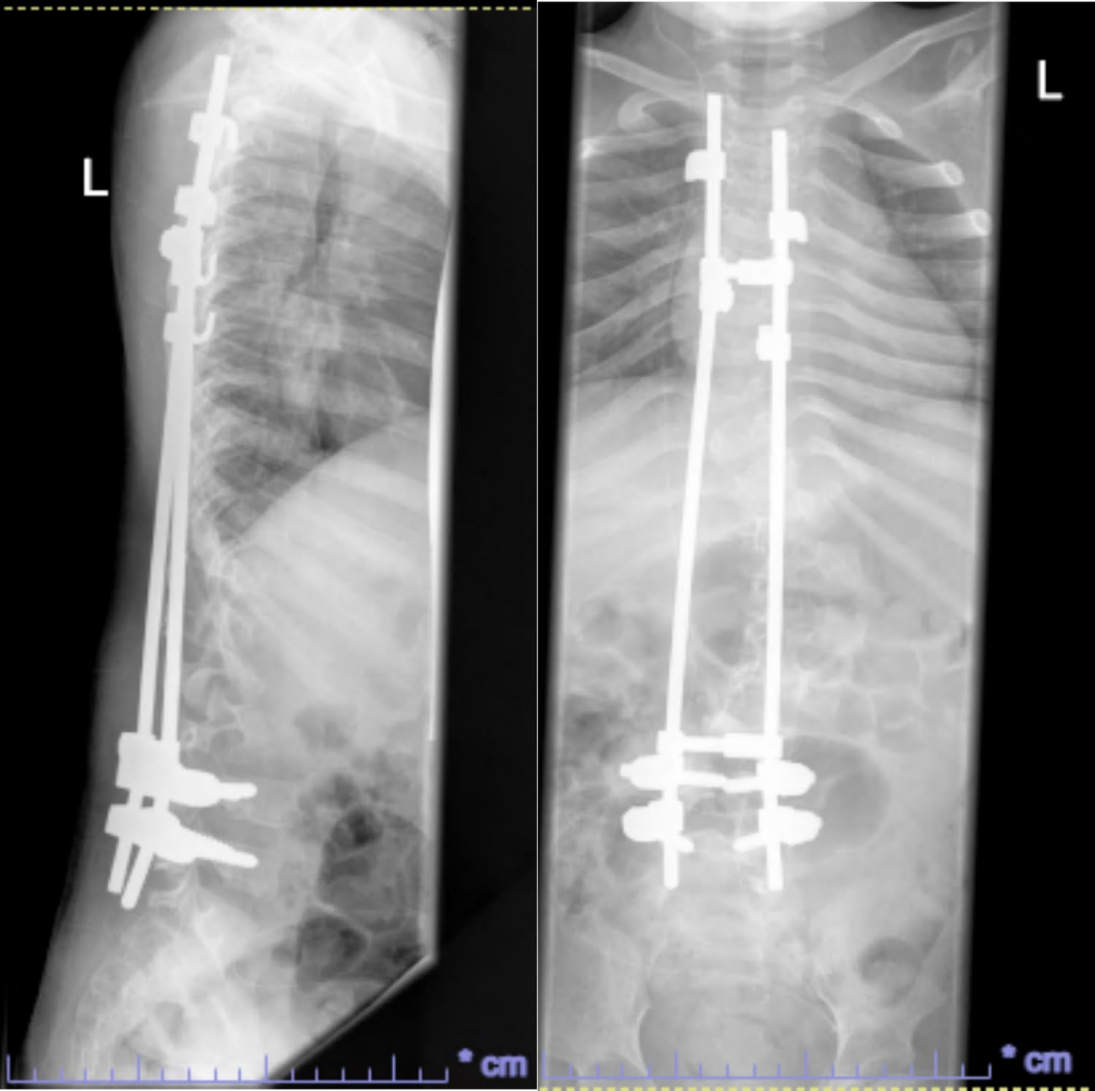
Post operative X-ray after implantation of TGR, aged 5
